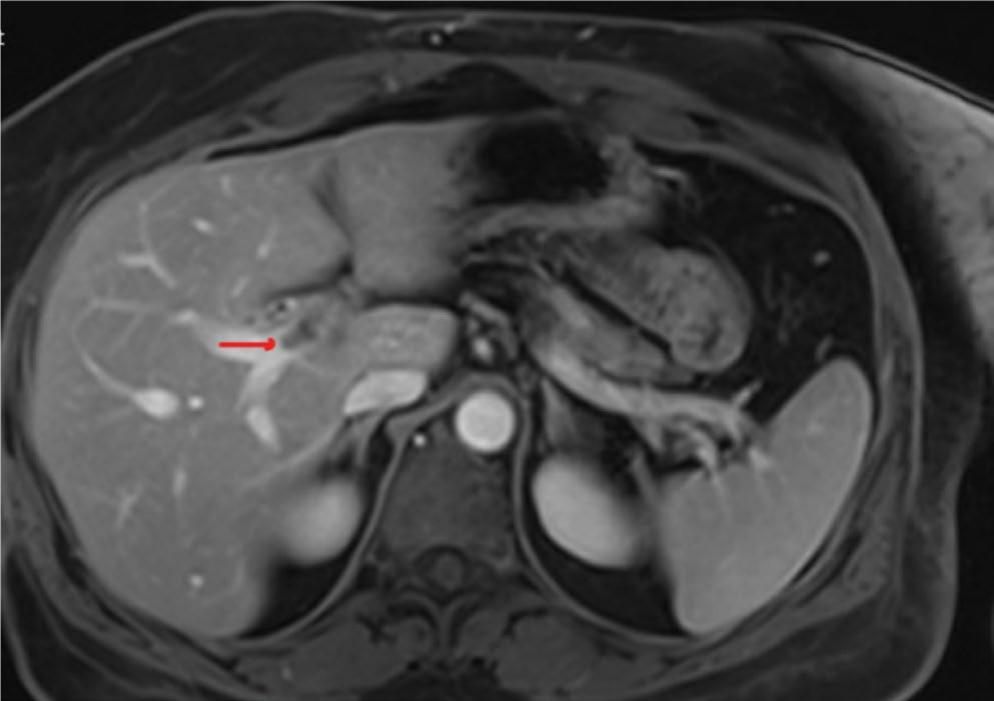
MRI scan of the abdomen showing the left renal vein thrombosis.
